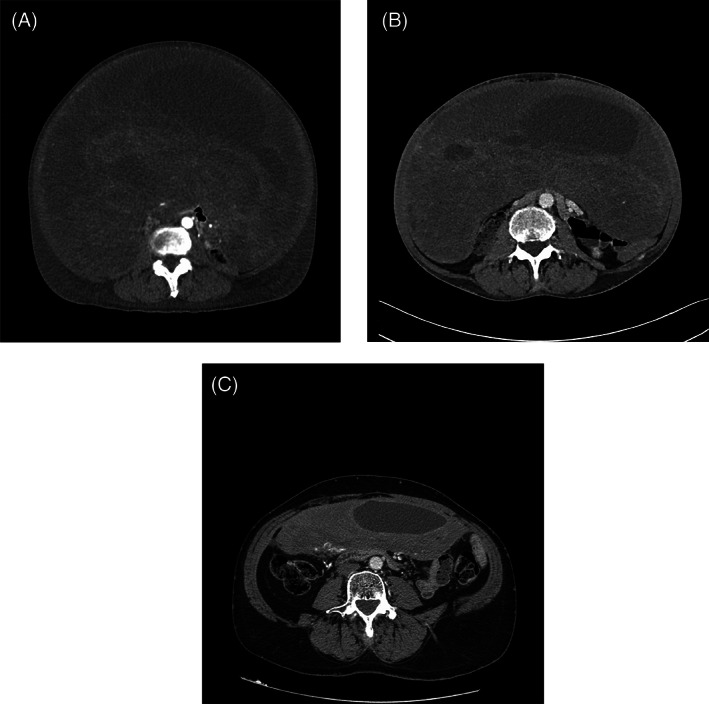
Case 3. (A) Contrast‐enhanced CT scan, at the onset of severe complication (35 × 22 cm). (B) DF shrinkage (30 × 12 cm) after 14 weekly cycles of doxorubicin at the discharge. (C) The last CT scan showing further reduction of the DF (23.5 × 6.5 cm), 6 months after the discontinuation of the chemotherapy [Correction added on 12 July 2021, after first online publication: In the original published version, Figures 1 and 2 have been corrected the size in this version].
